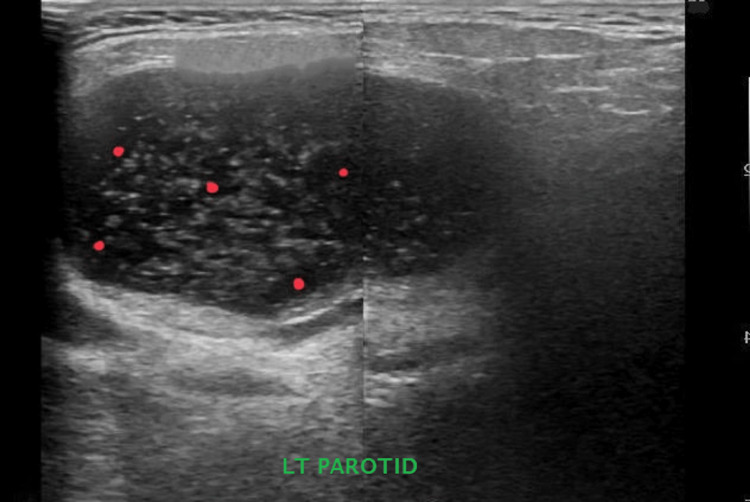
Ultrasound of the left parotid gland showing the presence of multiple variable-sized cystic areas (red dots) in the superficial and deep lobes of the gland, with internal striations favoring benign lymphoepithelial lesions of the left parotid gland. LT: left
